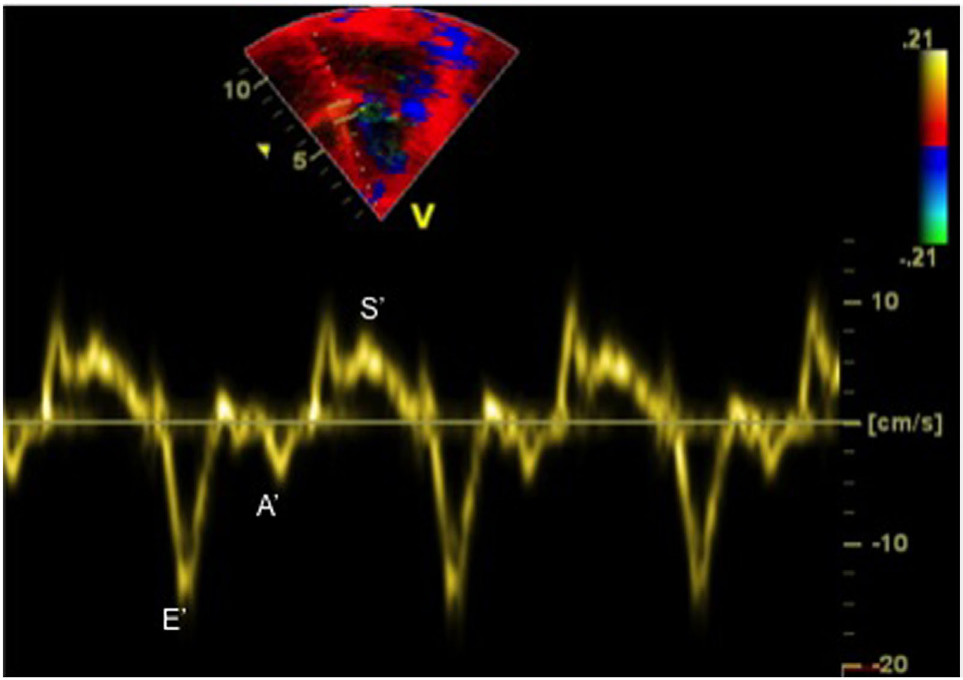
Tissue Doppler imaging tracing obtained at the septal annulus showing S′ (systolic) wave, E′ (early diastolic) wave, and A′ (late diastolic related to atrial contraction) wave.
